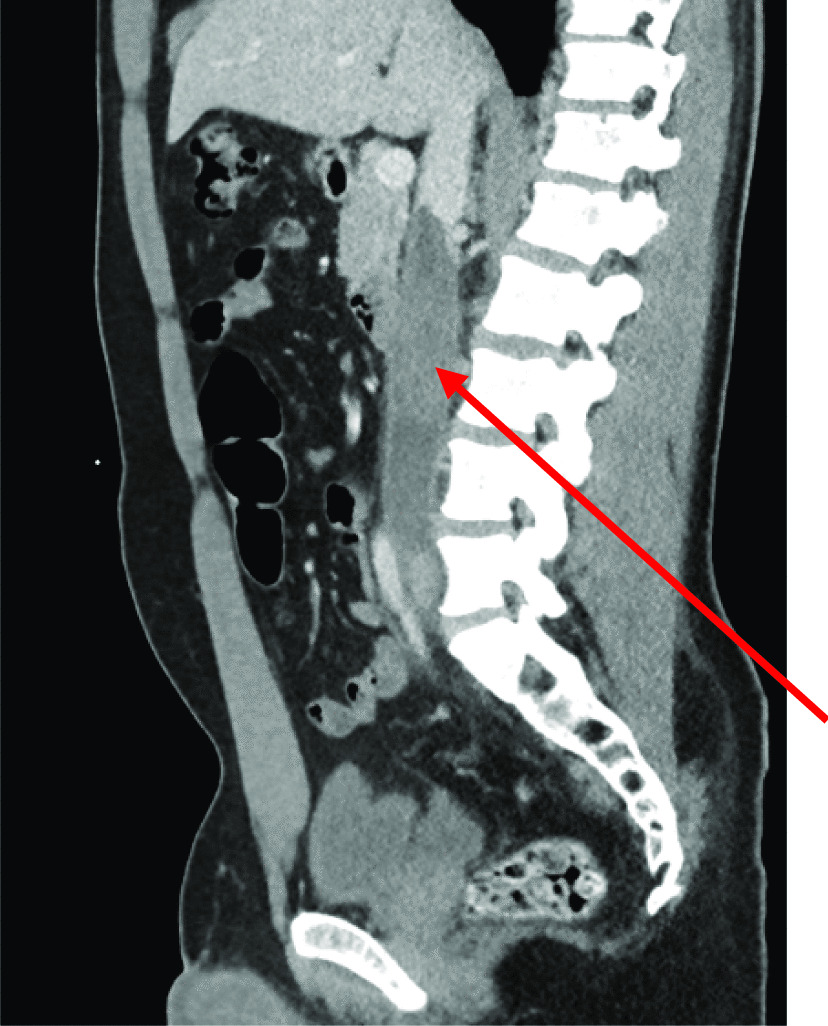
CT abdomen showing extensive thrombus (arrow) extending from the left common iliac vein through the IVC at the level of the renal veins
